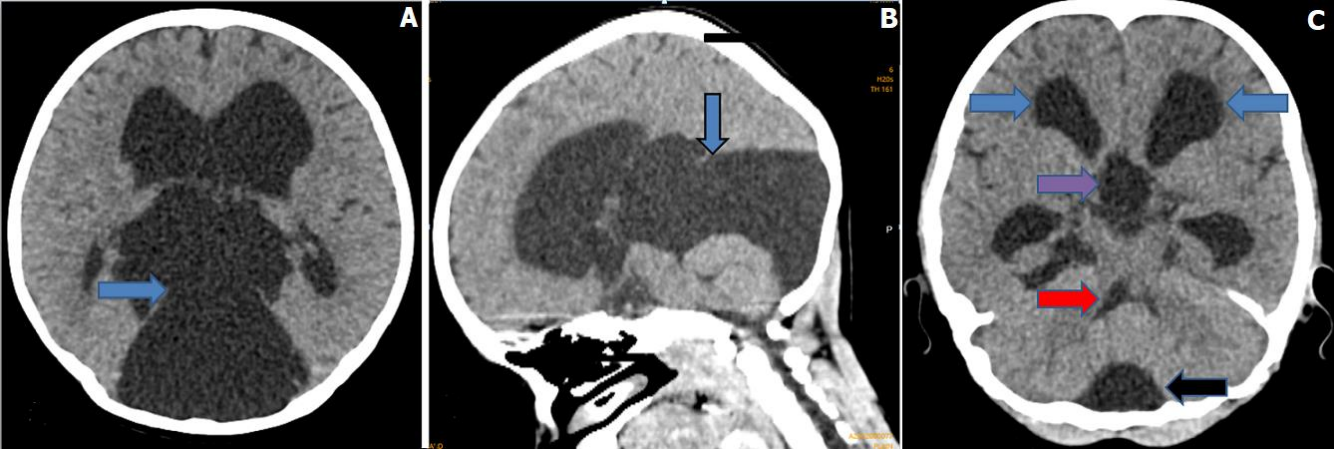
computed tomography scan axial section (A) and sagittal section (B) brain window showing retrocerebellar cyst communicating with quadrigeminal cistern with dilatation of bilateral lateral ventricle and third ventricle; (C) computed tomography scan axial section brain window showing retrocerebellar cyst (black arrow), dilatated bilateral lateral ventricle (blue arrow) and third ventricle (purple arrow), note the non-dilated fourth ventricle (red arrow)
